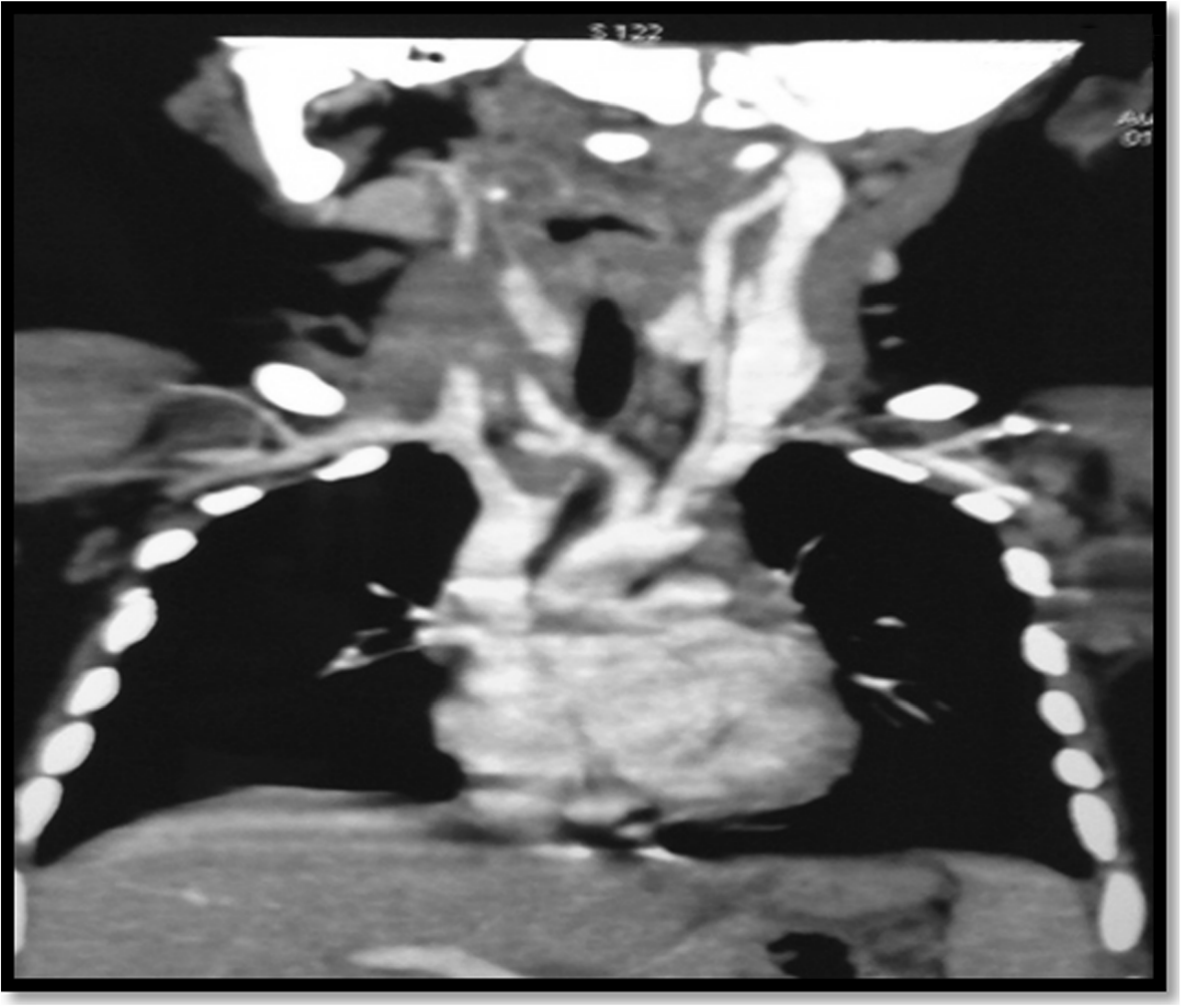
Contrast-enhanced computed tomography of the neck and chest showing a highly vascular heterogenous soft tissue lesion, pushing the neck vessels posteriorly with no retrosternal extension
